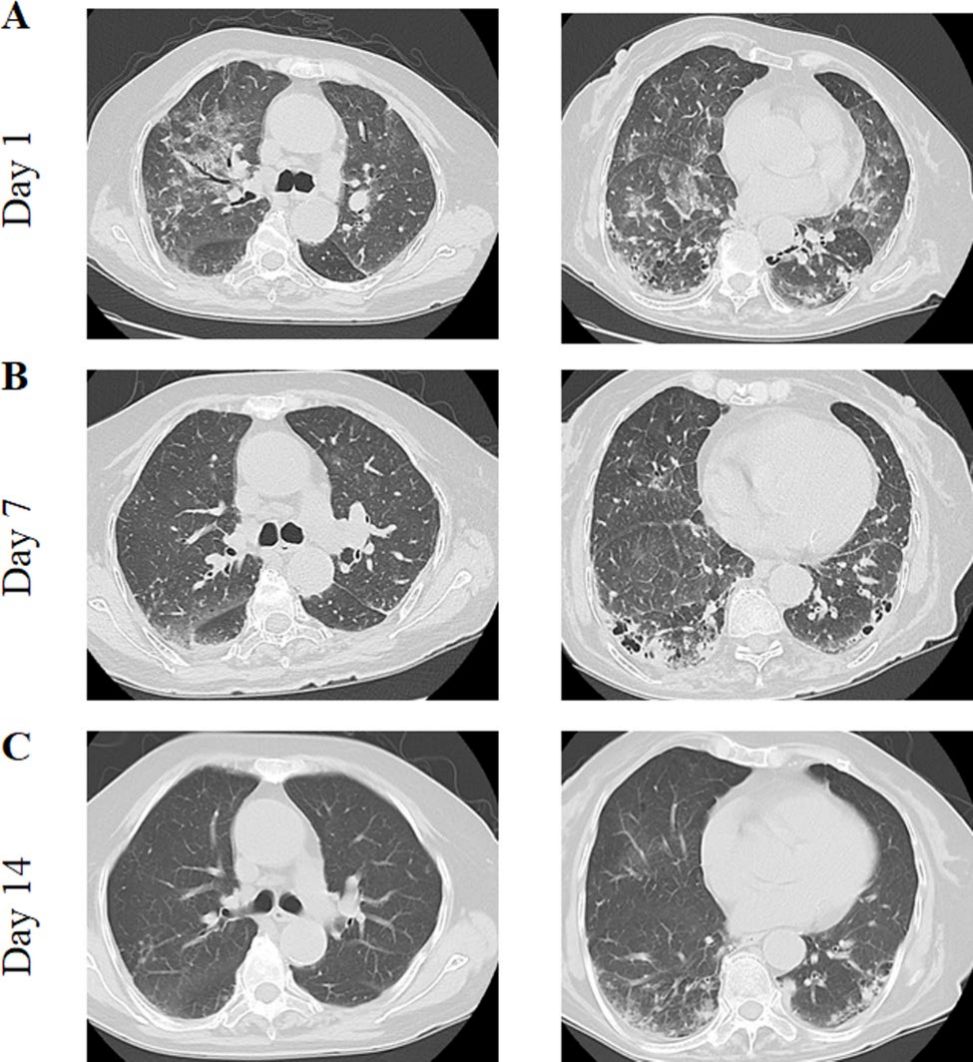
Case 4-Computed tomography images at different hospital days
